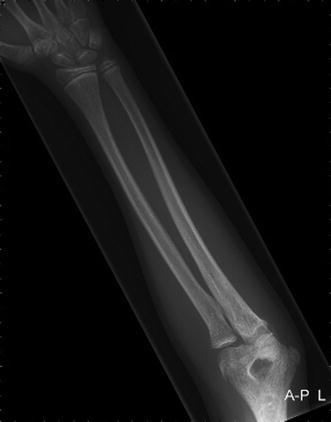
AP radiograph of the unaffected left forearm
